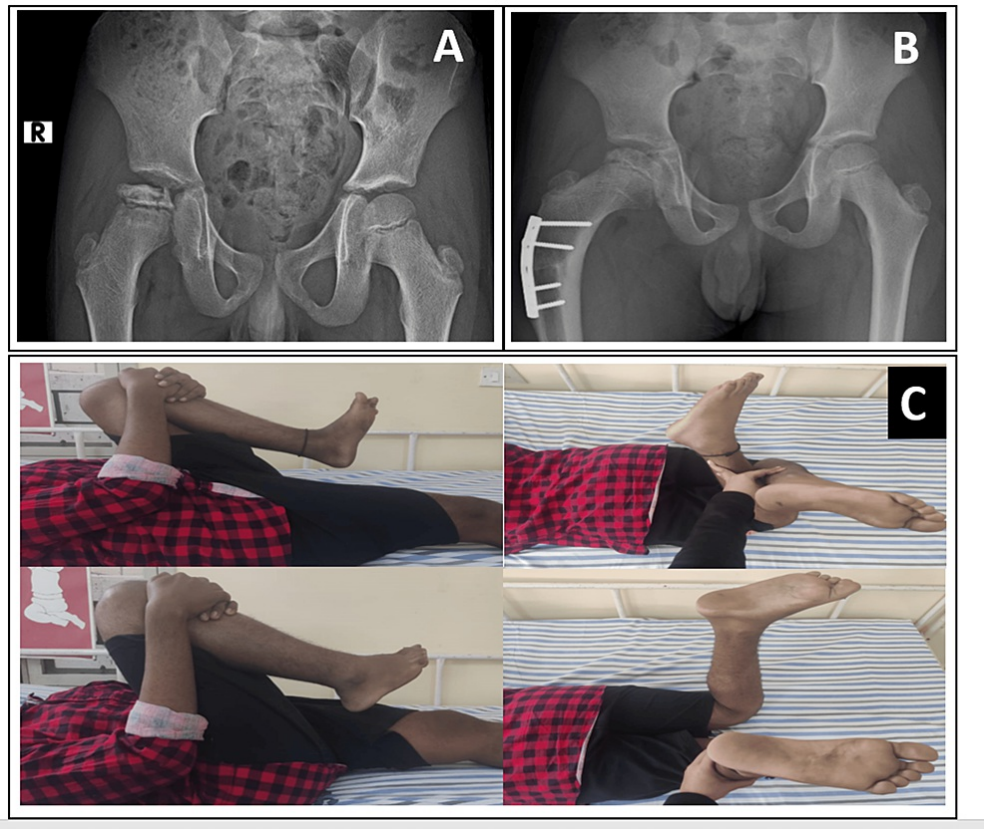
A 10-year-old boy with LCPD. (A) Preoperative radiograph showing modified Elizabethtown Stage IIB. (B) Postoperative radiograph taken at a follow-up of two years. (C) Range of movement found satisfactory at a follow-up of two years. LCPD: Legg-Calve-Perthes Disease.
